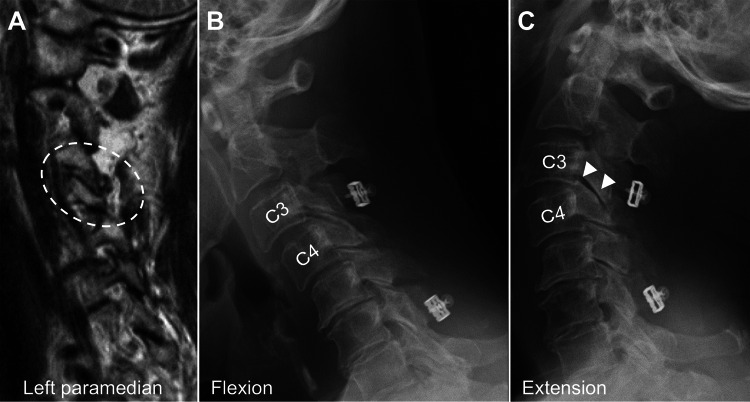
Postoperative cervical radiological images. (A) Left paramedian sagittal T2-weighted magnetic resonance imaging demonstrating the disappearance of interarticular fluid intensities in the C3-4 facet joint (dashed circle). (B, C) Dynamic radiographs showing unchanged C3-4 vertebral body translation and smaller facet joint shadows during extension compared with preoperative images (arrowheads).
